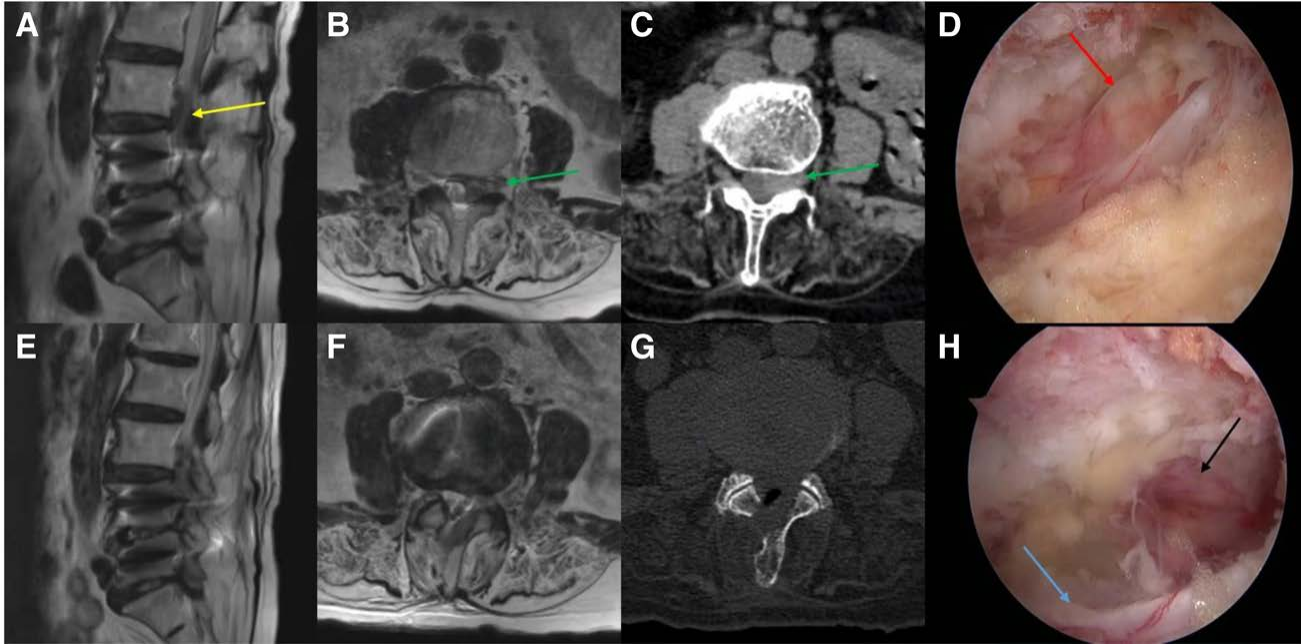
(A) Preoperative sagittal MRI showed an up-migrated herniated disc at the L3–L4 level (yellow arrow), (B, C) axial MRI and CT showed that the herniated disc was partially intruding in the foraminal area (green arrow), (D) the up-migrated herniated disc (red arrow) was exposed, (E, F) Postoperative sagittal and axial MRI revealed that the herniated disc was removed, and nerve roots were decompressed, (G) axial CT showed that the facet joint was less involved, and (H) the exiting (black arrow) and traversing (blue arrow) nerve roots were completely decompressed. CT = computed tomography, MRI = magnetic resonance imaging.
